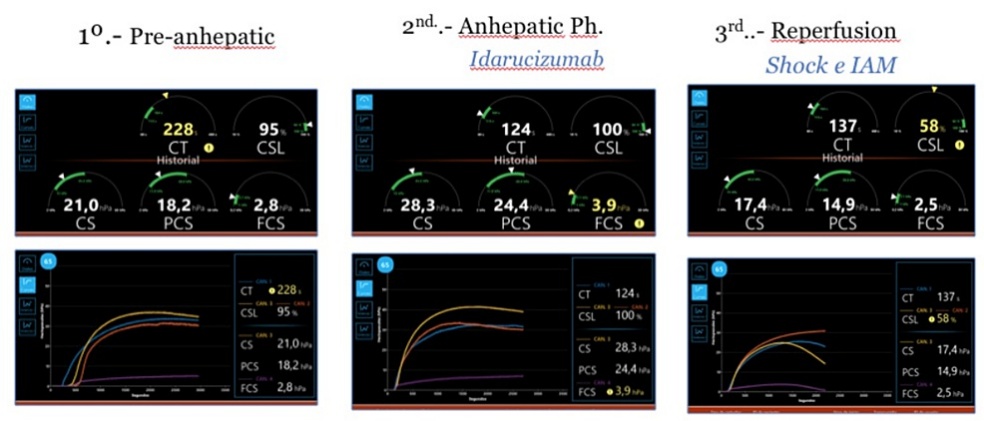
Viscoelastic hemostatic assay The image shows the Quantra® System, which is based on SEER (Sonic Estimation of Elasticity via Resonance) sonorheometry. Images show graphically the information shared in Table 1. The left images represent the study before the surgical incision. Images at the center show the mechanical properties of the clot after the use of idarucizumab. The right images aim to show the hemostasis status after the reperfusion phase. CT: clot time; CSL: clot stability to lysis; CS: clot stiffness; PCS: platelet contribution to clot stiffness; FCS: fibrinogen contribution to clot stiffness.
